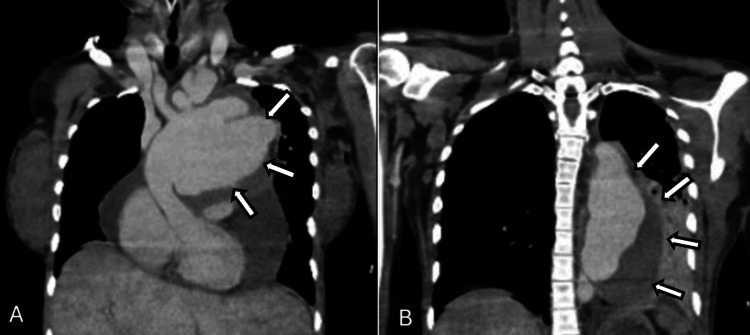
Preoperative CT angiography showing extensive pseudoaneurysm of the aortic arch (A) and descending aorta (B) CT: computed tomography
